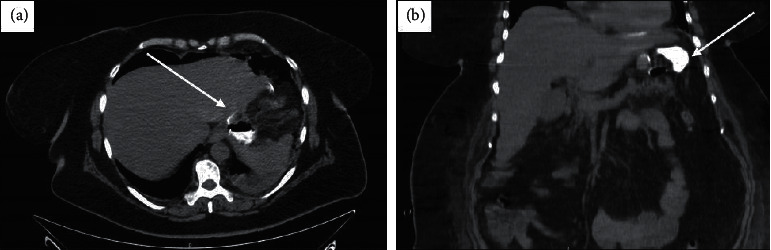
The early complicated patient, shown on axial (a) and coronal (b) CT-scan during the portal phase. CT images show the GL and corresponding collection on the 2^nd^ POD (Figure 2(a), white arrow). The gastric fistula linking the stomach and enhanced collection (white arrow) are shown.
